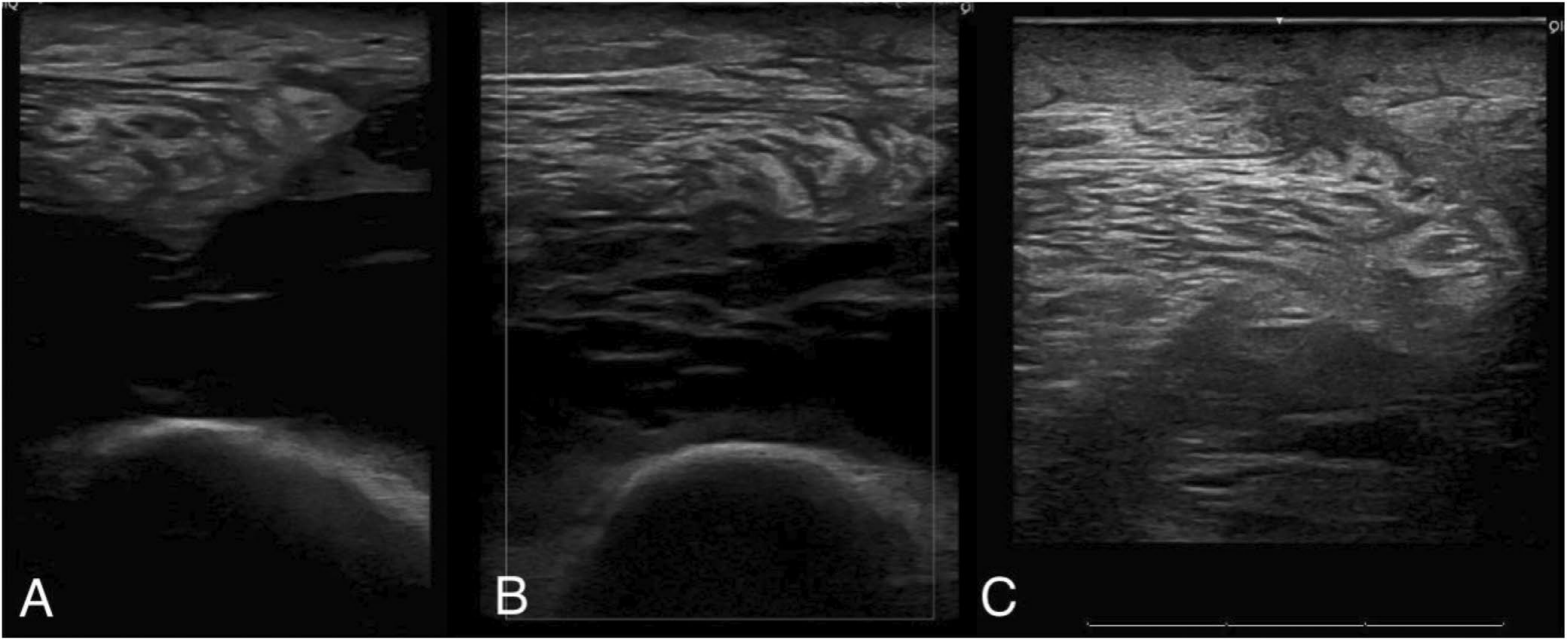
(A) Ultrasound done at postoperative follow‐up on August 10, 2023. (B) Ultrasound done at postoperative follow‐up on August 31, 2023. (C) Ultrasound done at postoperative follow‐up on November 13, 2023. Postoperative seroma is clearly visualized. Over the course of time, seroma resolution is visible, as is tissue regeneration.
